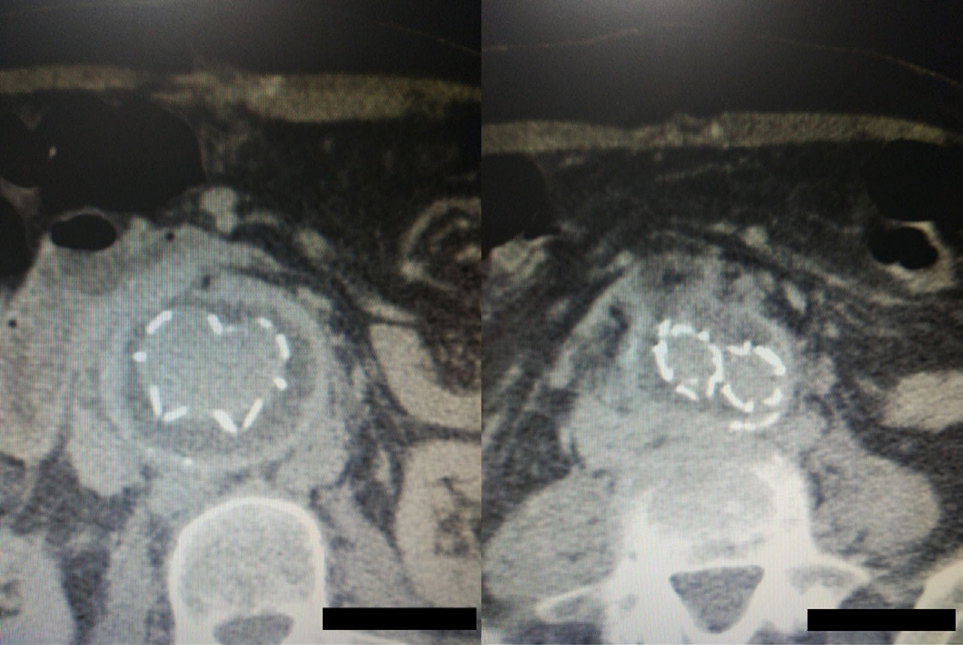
Postoperative computed tomography angiography images showing the graft wrapped by the sac without any sac enlargement or obvious endoleak.
